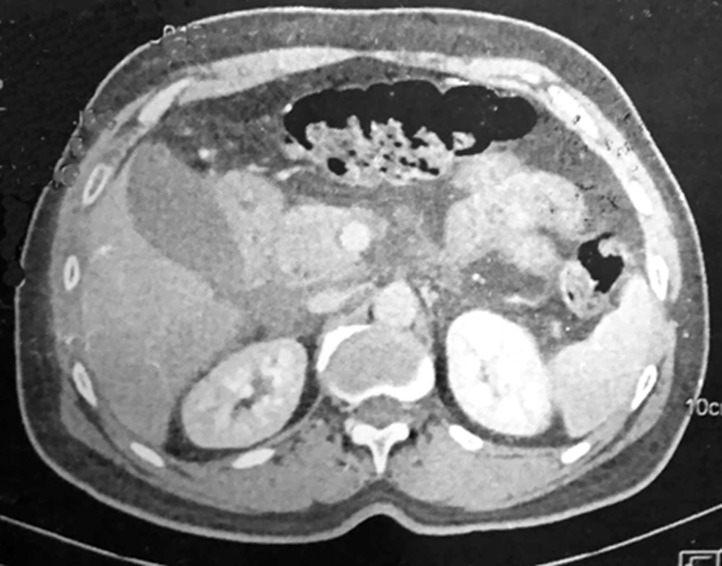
preoperative computed tomography showing intra-abdominal fluid and infiltration of the epigastric fat extending to the hepatic hilum and the root of the mesentery
